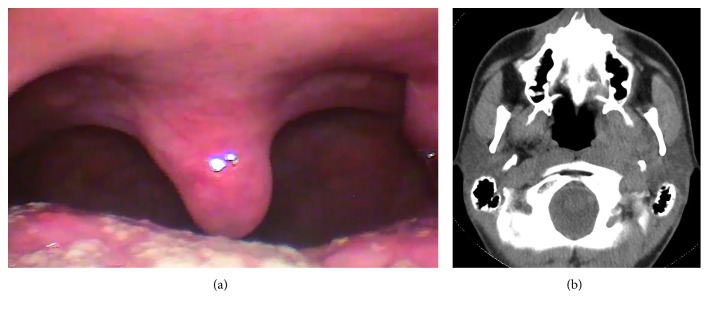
(a) Normal contour of the oropharynx six months postoperatively. (b) Computed tomography axial image: no tumor relapse is seen.
